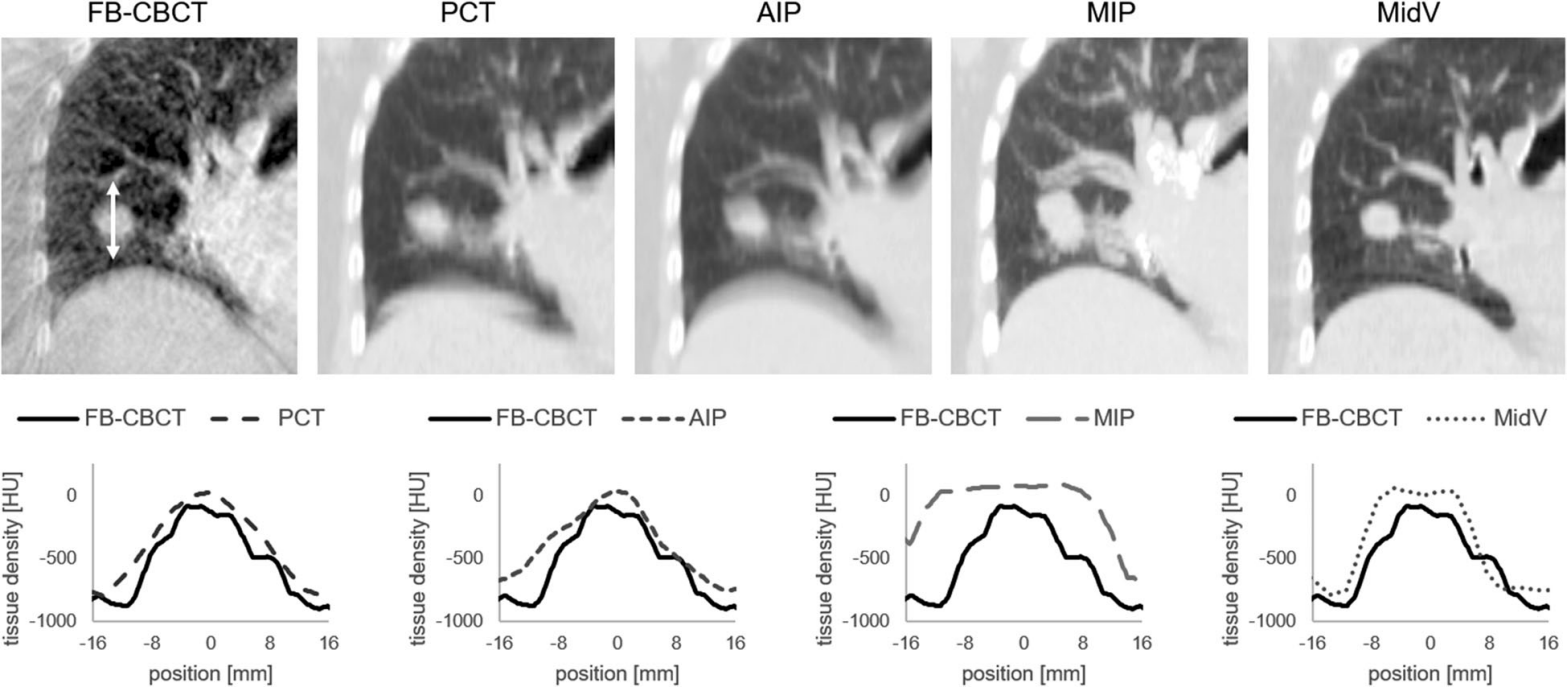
Tumor depiction in the different CT datasets. The visibility of the tumor of a lung patient in the CT datasets is presented in the upper part. Line profiles (lower part) show the density variation of the tumor in z-direction (superior-inferior) in the CT datasets in comparison to FB-CBCT. FB-CBCT = free breathing cone beam CT; PCT = slow planning CT; AIP = average intensity projection; MIP = maximum intensity projection; MidV = mid-ventilation
